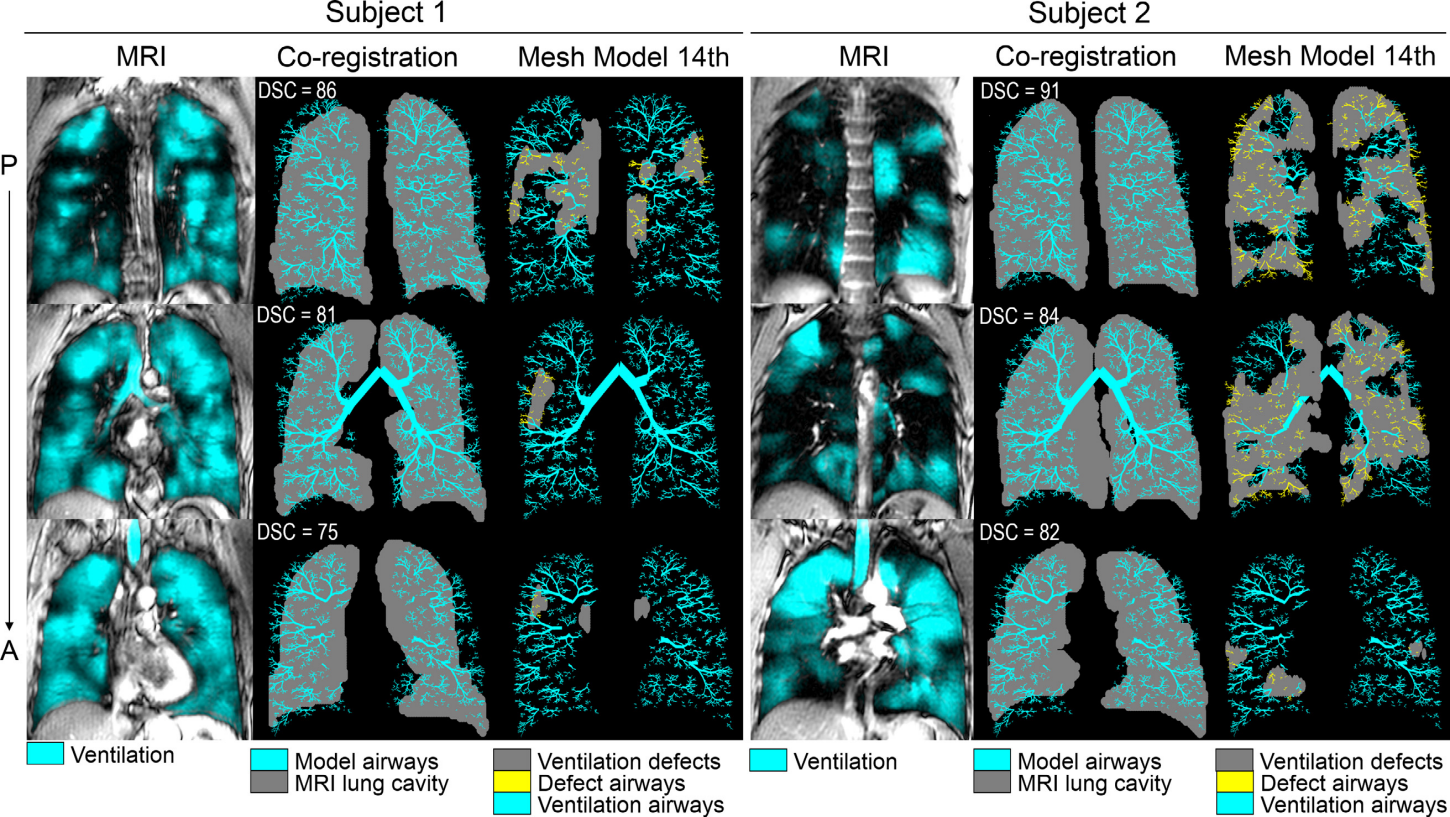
Coregistration of MRI and airway mesh model for three center slices in two representative asthmatics. For Subject 1 and 2, left panels show center, and 2 slices anterior (A) and posterior (P) to center coronal slice MRI; middle panels show corresponding model‐to‐MRI lung cavity coregistration; right panels show coregistration of model‐to‐ventilation defects coregistration with 14th generation airways proximal to ventilation defects closed in yellow. Slice‐specific Dice similarity coefficients (DSC) reflect registration accuracy for each of the three slices coregistered to the airway model for each of Subject 1 and 2.
